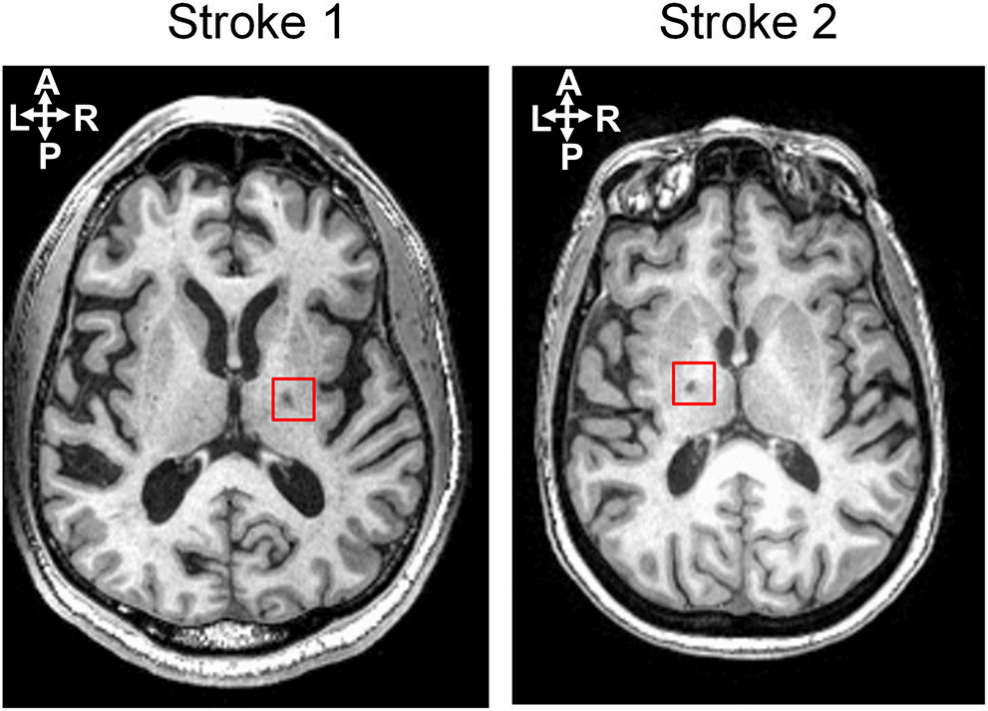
Lesion locations for two stroke subjects shown on an axial slice of the T1-weighted anatomic images.
